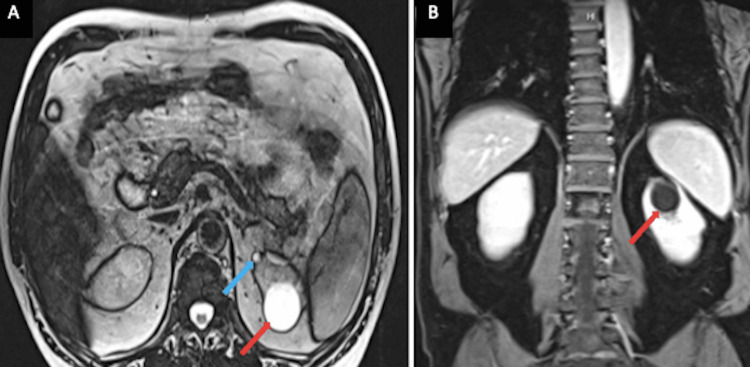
MRI of the abdomen MRI of the abdomen in cross-sectional (Panel A) and longitudinal (Panel B) view showed a benign-appearing simple left renal cyst (red arrows) along with another tiny simple cyst measuring 9 mm more anteriorly in the upper pole of the left kidney (blue arrow in panel A).
